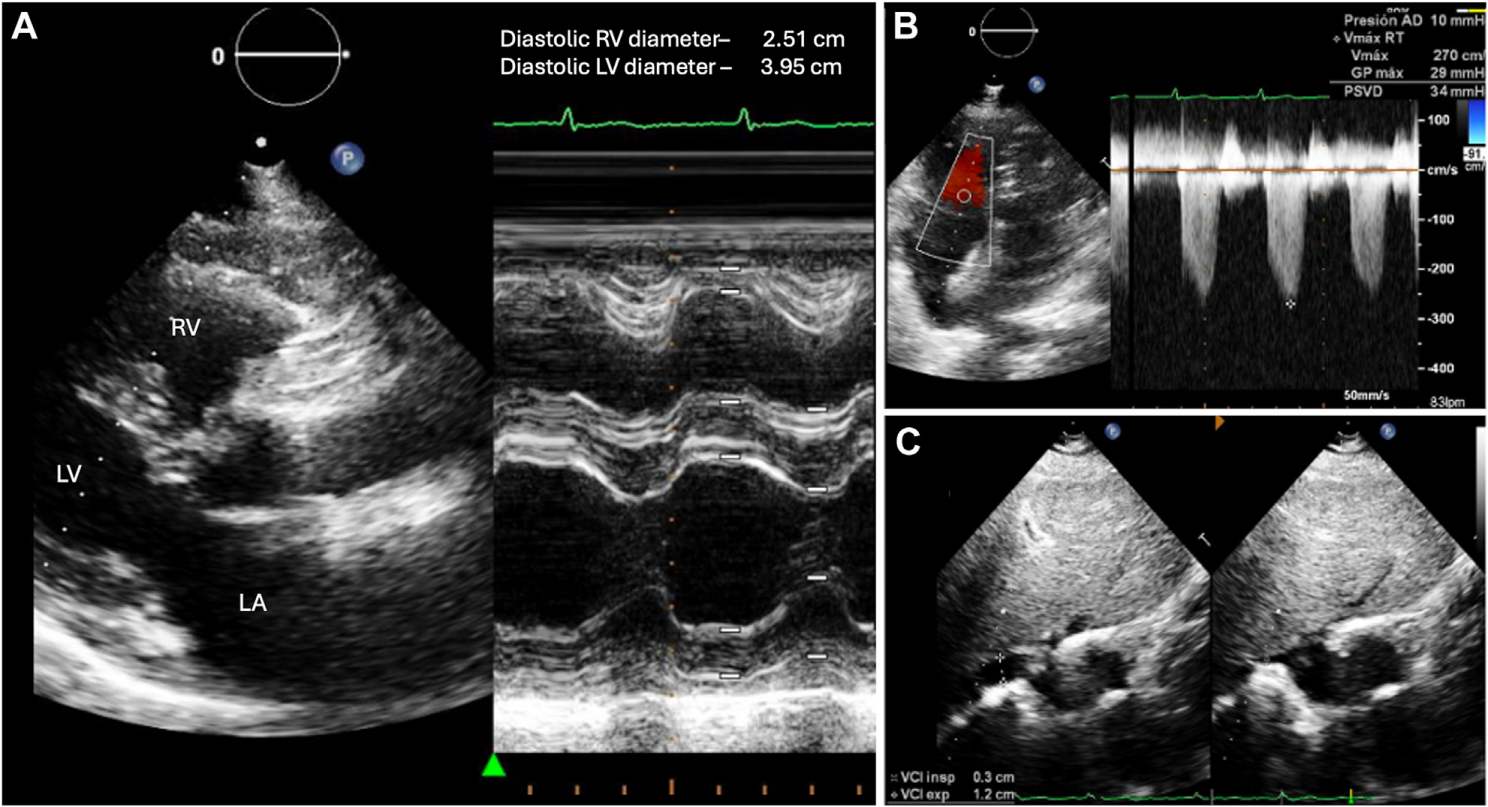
Two-dimensional TTE-guided M-mode, parasternal long-axis view (A) 24 months post-ASD closure, demonstrates normal RV size and interventricular septal motion. Right ventricle–focused apical 4-chamber view (B) with color-flow Doppler-guided continuous-wave Doppler reveals a peak TR velocity of 2.7 m/sec and normal estimated RV systolic pressure. Subcostal view (C) demonstrates a normal diameter of the inferior vena cava with normal inspiratory collapse. *LA*, Left atrium; *LV*, left ventricle; *RV*, right ventricle.
